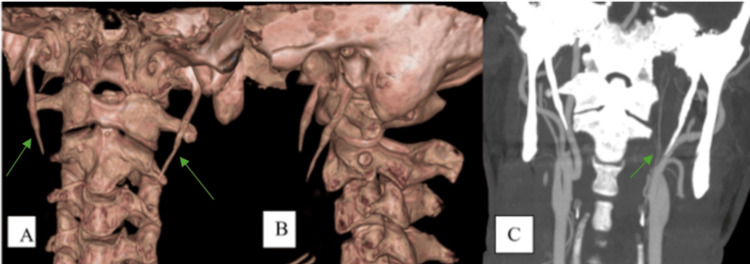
Eagle syndrome on CT angiography (CTA) with three-dimensional reconstruction (A) Anterior 3D volume-rendered CTA reconstruction demonstrating bilateral elongated styloid processes (~50 mm; normal <25-30 mm) projecting inferiorly from the skull base (arrows). (B) Oblique 3D volume-rendered reconstruction showing the elongated styloid processes in profile, coursing inferiorly toward the cervical carotid space. (C) Coronal CTA demonstrating the elongated styloid processes adjacent to the cervical internal carotid arteries (ICAs); the left ICA is non-opacified at the level of styloid contact (arrow), consistent with dissection and occlusion.
